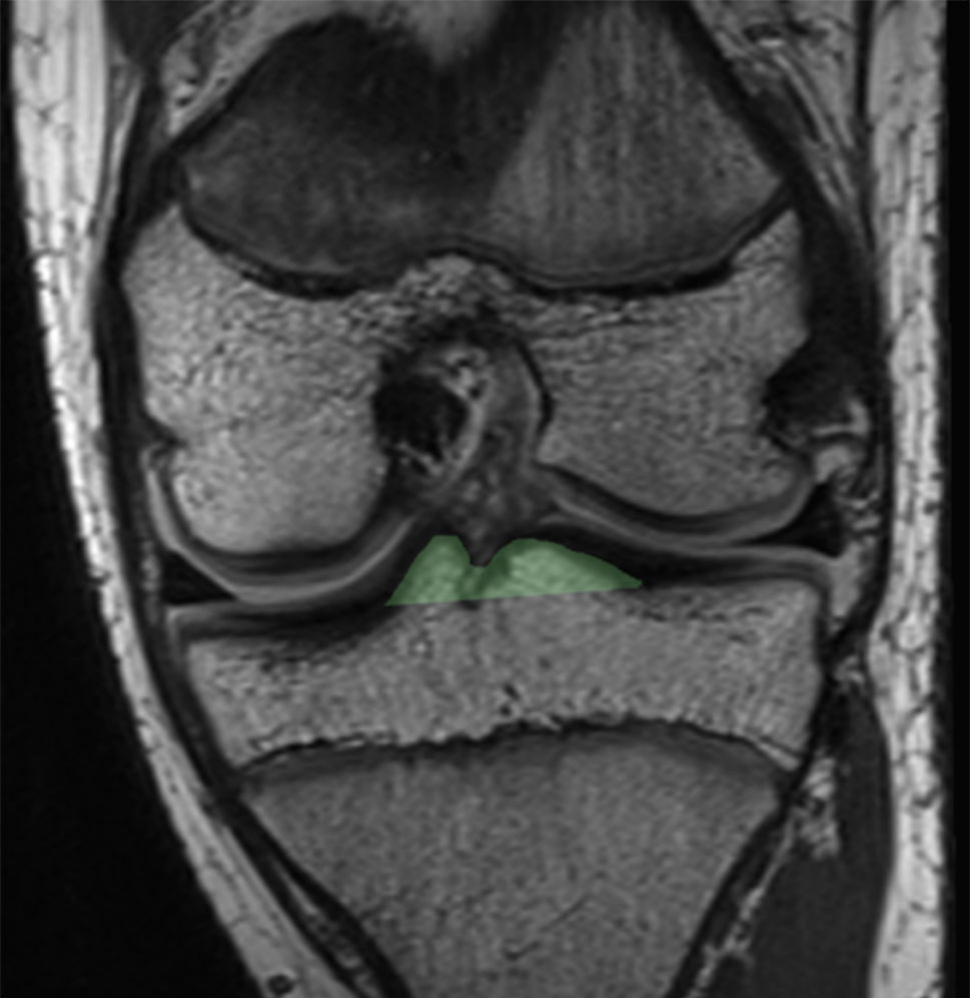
A single coronal image of a proton density (PD) magnetic resonance imaging (MRI) sequence demonstrating the measuring technique for the tibial eminence volume. The measuring technique was applied to all coronal slices of the tibial eminences and summated to generate the volumetric data
